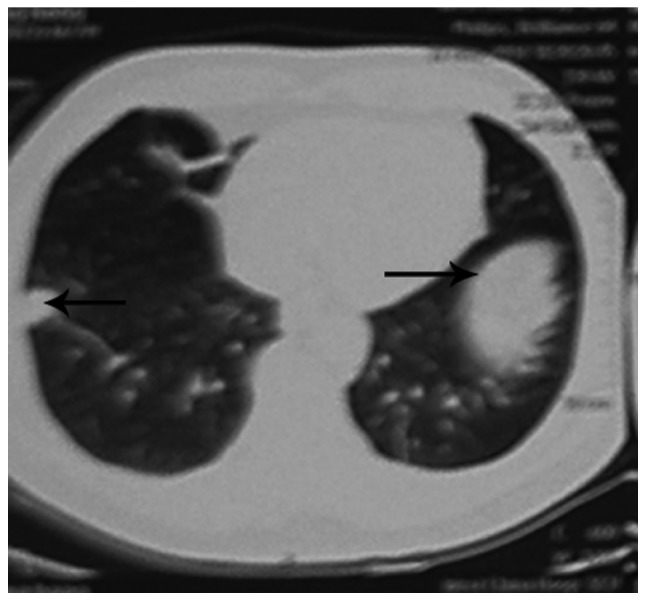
Chest radiograph of one patient showing metastases in the bilateral lower lobes of the lung (black arrows).
